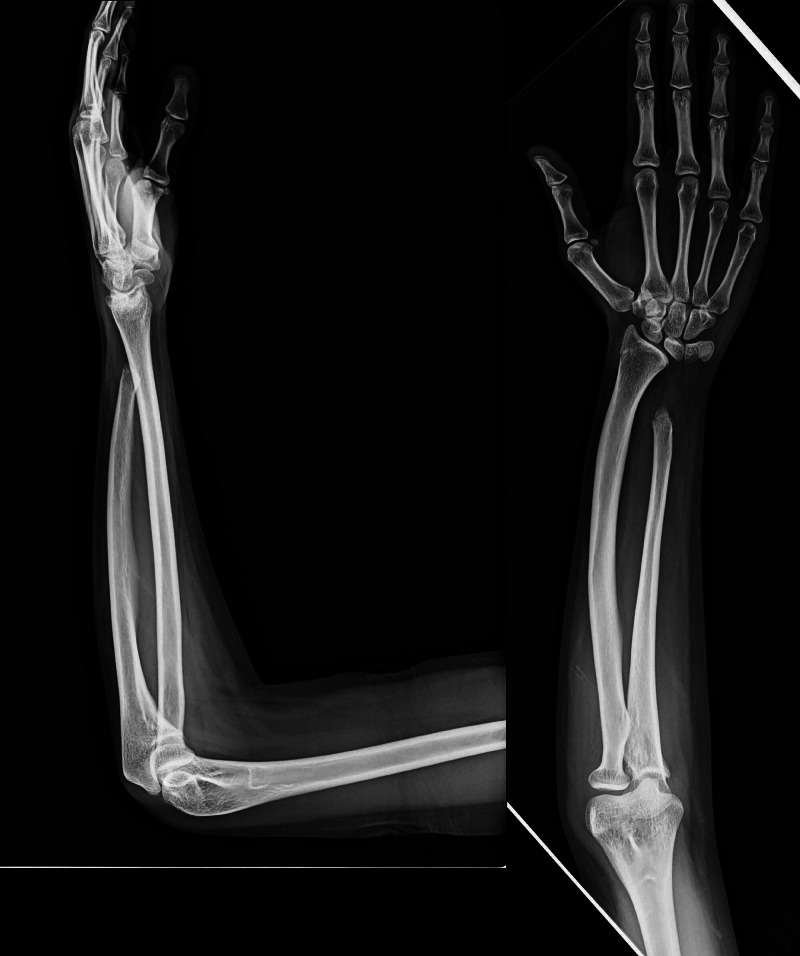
Radiographs show new variant type I congenital ulna deficiency right forearm and wrist Radiographs show a new variant type I congenital ulna deficiency right forearm and wrist of an 18-year old girl at the time of skeletal maturity. The posteroanterior and lateral views show ulna hypoplasia and radius bowing with normal hand, five digits, and normal elbow joint.
